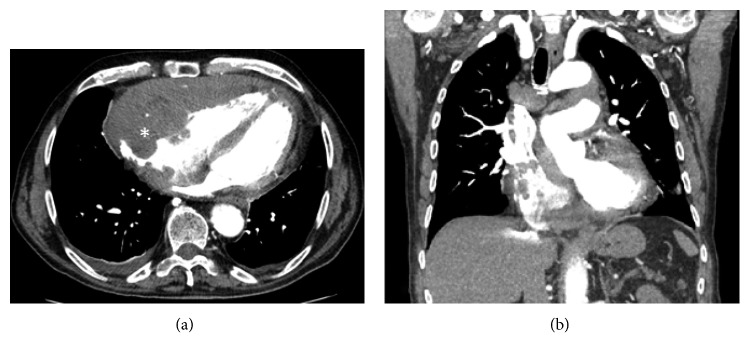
Computed tomography chest with pulmonary embolism protocol. (a) Transverse view and (b) coronal view. In the transverse view, there is extensive involvement of the anterior portion of the cardiac tissue and that encases the right atrium, right ventricle, and the great vessels (^∗^). There is evidence of filling defect in both the transverse and coronal views of the right atrium and ventricle, which suggests that the surrounding mass has infiltrated transmurally. The coronal view demonstrates how extensive the invasion is likely from an anterior to posterior approach. There is also extensive thickening of the transeptal and free wall.
